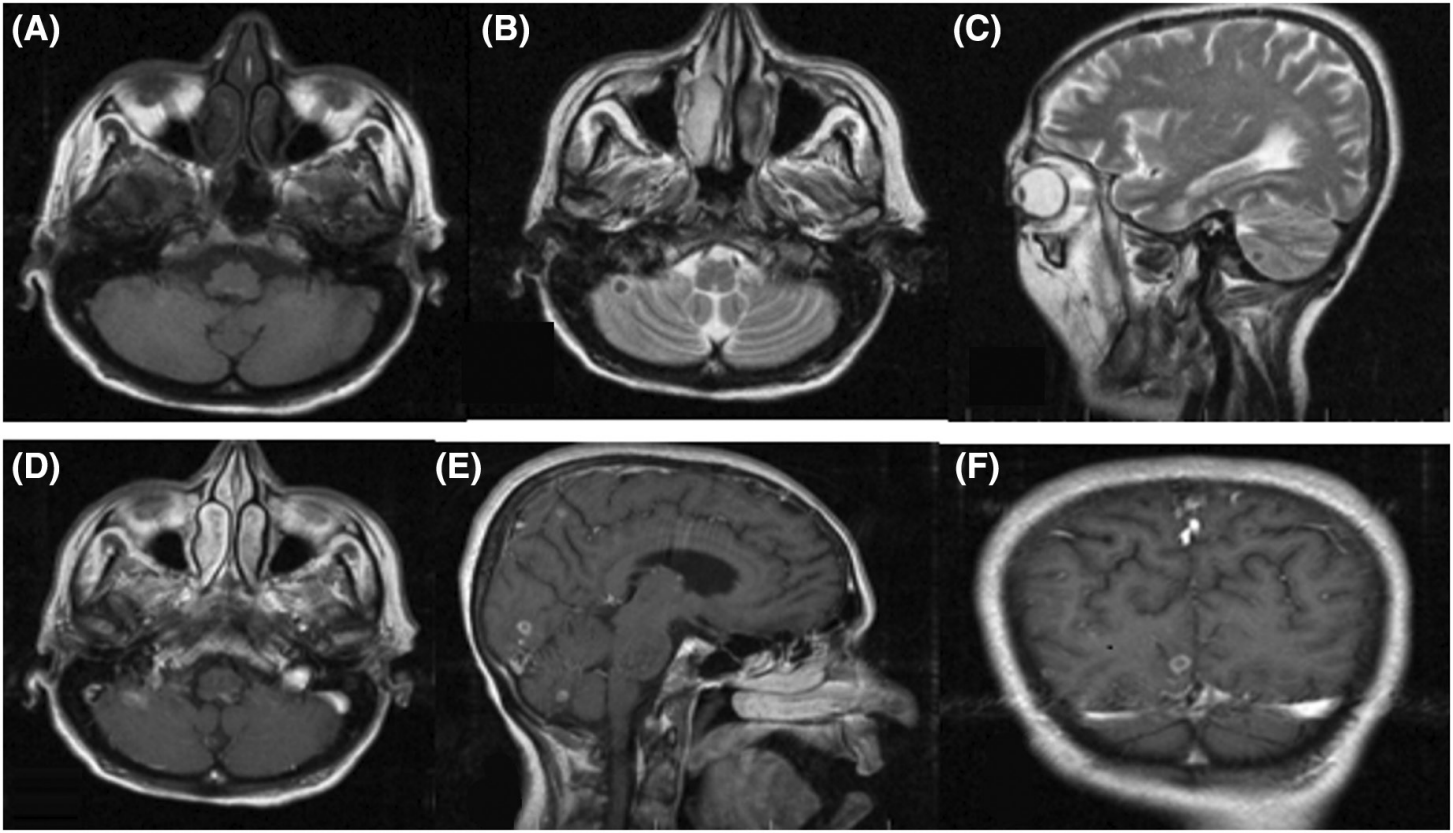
Brain MRI revealed multiple T1‐weighted hyposignal (4‐A) and T2‐weighted hypo/hypersignal (4‐B, 4‐C) lesions with ring‐like gadolinium enhancement in the cerebellum and throughout the cerebellum (4‐D, 4‐E, 4‐F), predominantly at the gray–white matter junction.
